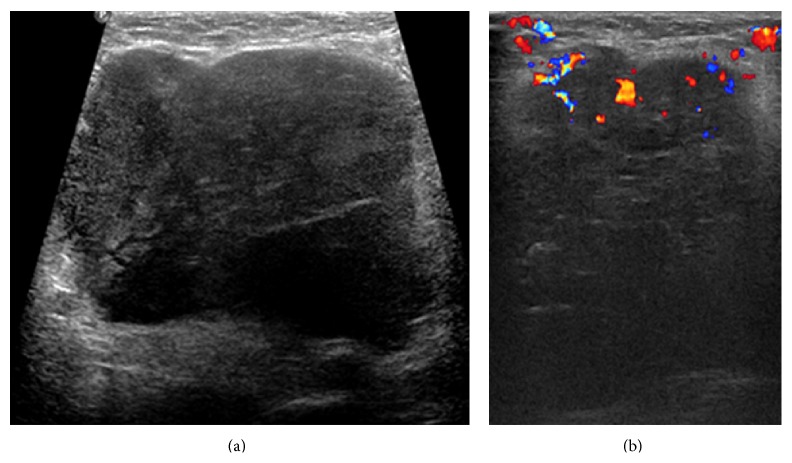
Transverse (a) and color Doppler (b) images of ultrasonography show an irregular mass with an indistinct margin, internal hypoechogenicity, and increased peripheral vascularity.
